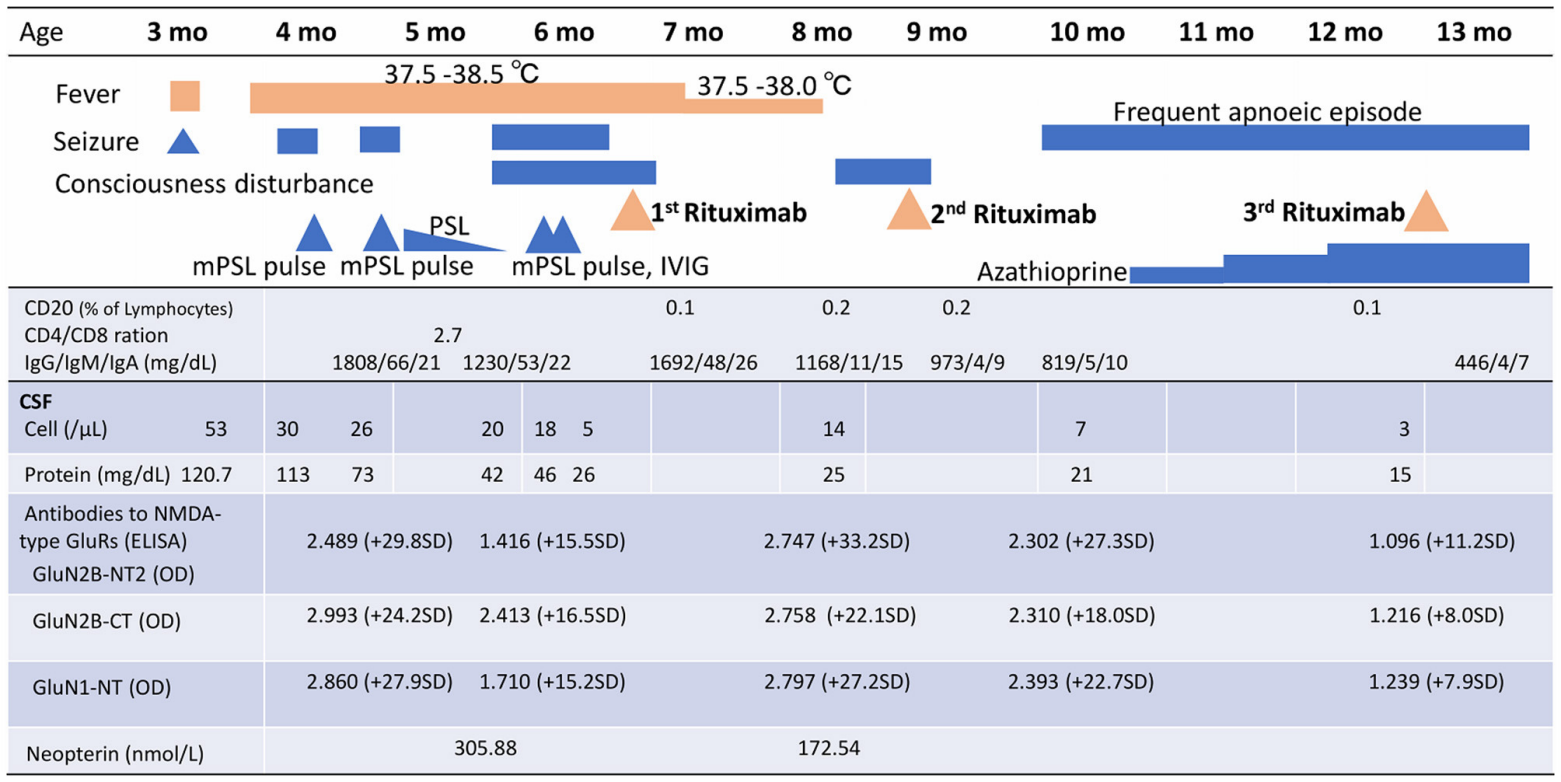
Clinical signs, treatment course, peripheral blood CD20^+^ B cells, and cerebral spinal fluid analysis, including anti-NMDAR antibody titres and neopterin, over time. mPSL, methylprednisolone; PSL, prednisolone; IVIg, intravenous immunoglobulin; CSF, cerebral spinal fluid; NMDA, N-methyl-D-aspartate; GluRs, glutamate receptors; ELISA, enzyme-linked immunosorbent assays; GluN2B-NT2, N-terminal of GluN2B; OD, optical density; GluN2B-CT, C-terminal of GluN2B; GluN1-NT, N-terminal of GluN1; SD, standard deviation.
